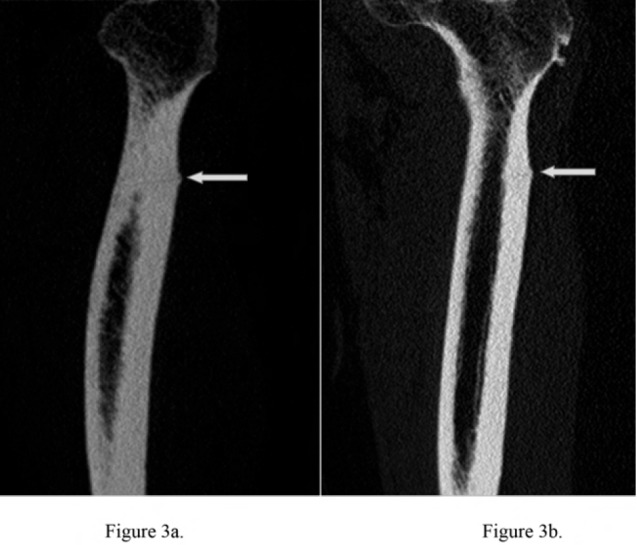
Figure 3a and 3b are reconstructed images from a limited multislice CT scan of the left femur showing a thin oblique linear
